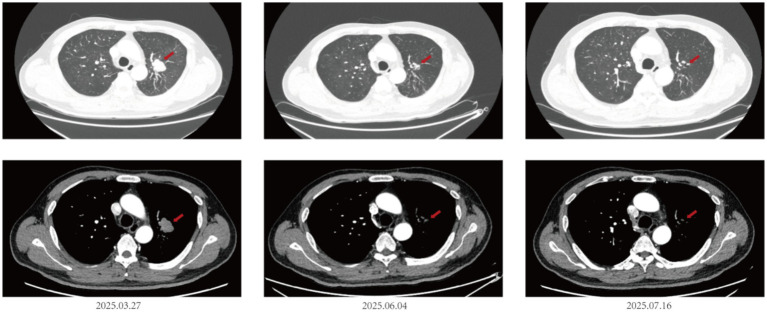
Evolution of chest CT findings during combination therapy in a patient with small cell lung cancer and brain metastases.
